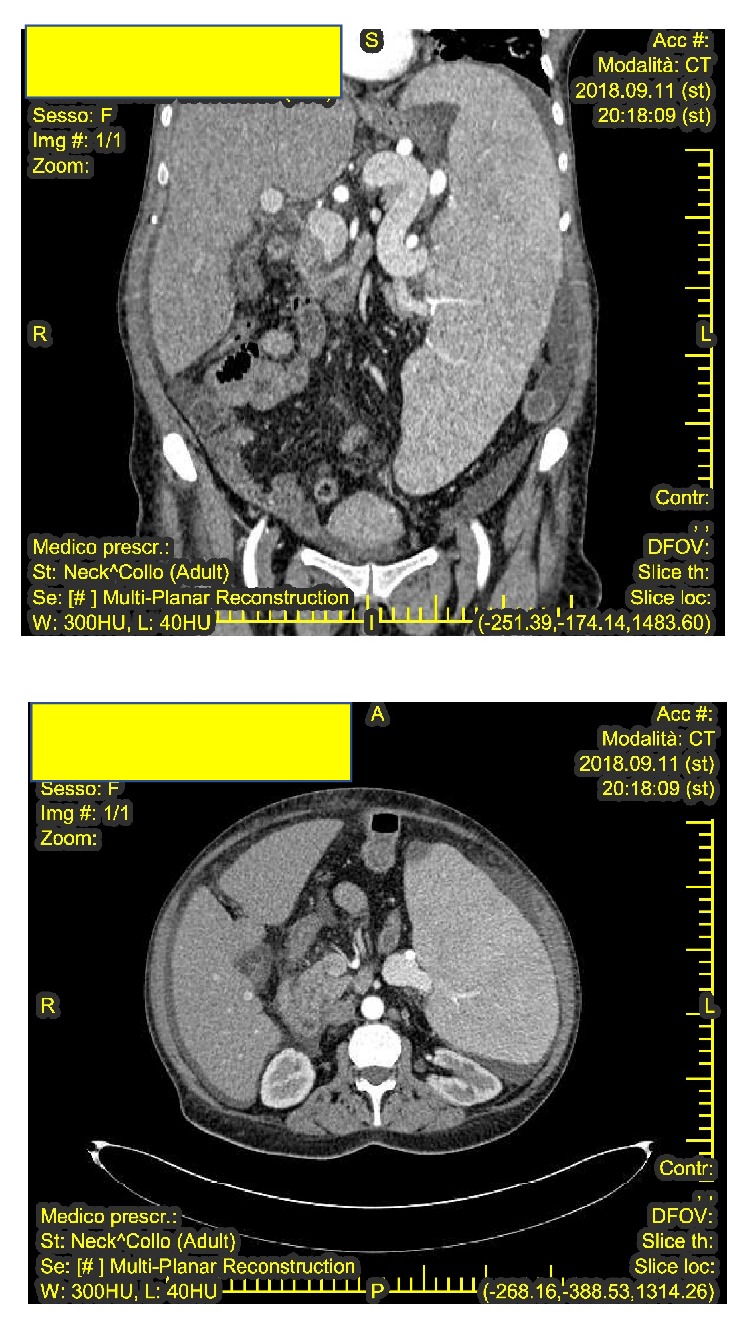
Thoracic -abdominal CT with contrast medium which confirmed the presence of adenopathy in the mediastinal space.
